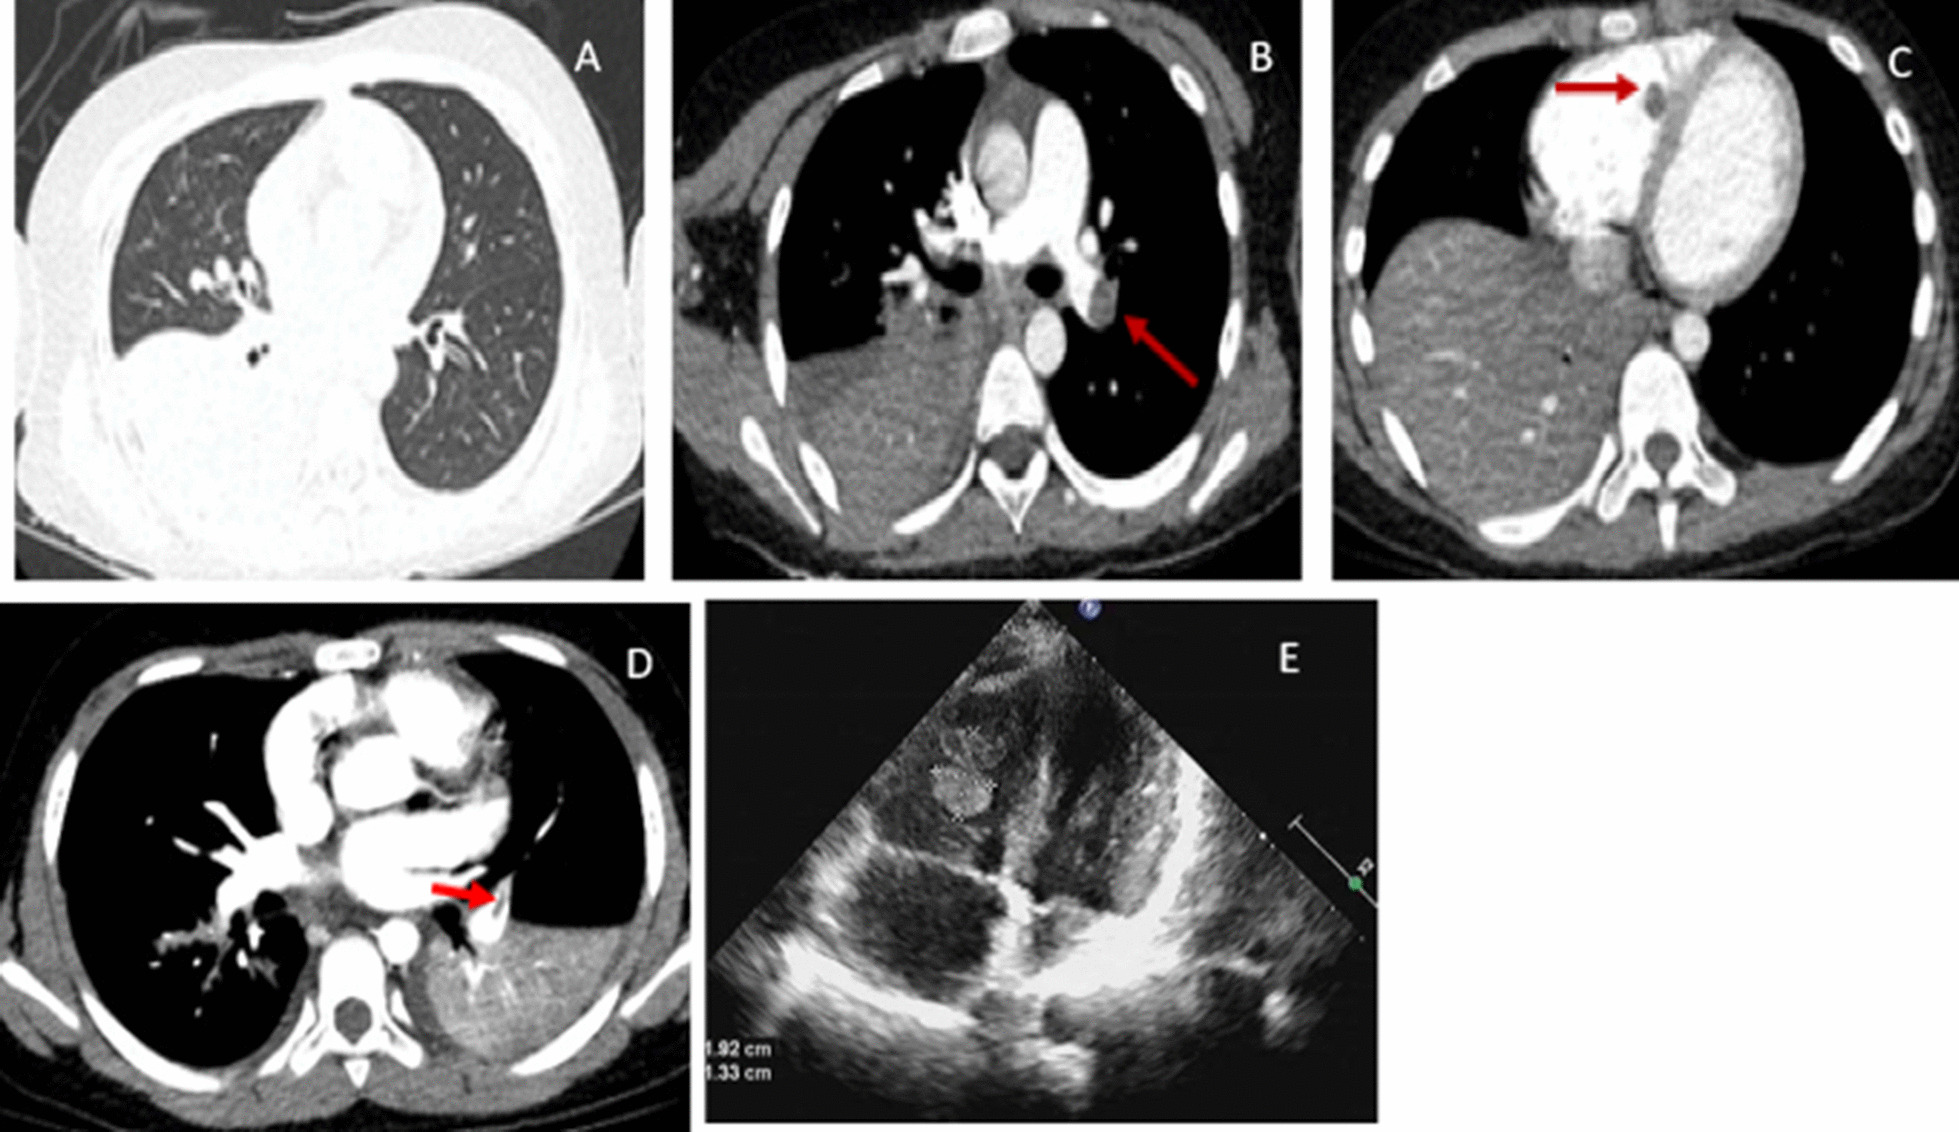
A Chest imaging revealed consolidation with high density in the right lower lobe. B, C Chest CTA showed filling defects in the left pulmonary artery and the right ventricle. D Coronal reconstruction of CTA shows filling defect of left pulmonary artery and right pleural effusion (arrow). E Echocardiography showed mass-like echo in the right ventricle, about 9 × 6 mm, 13 × 8 mm in size
